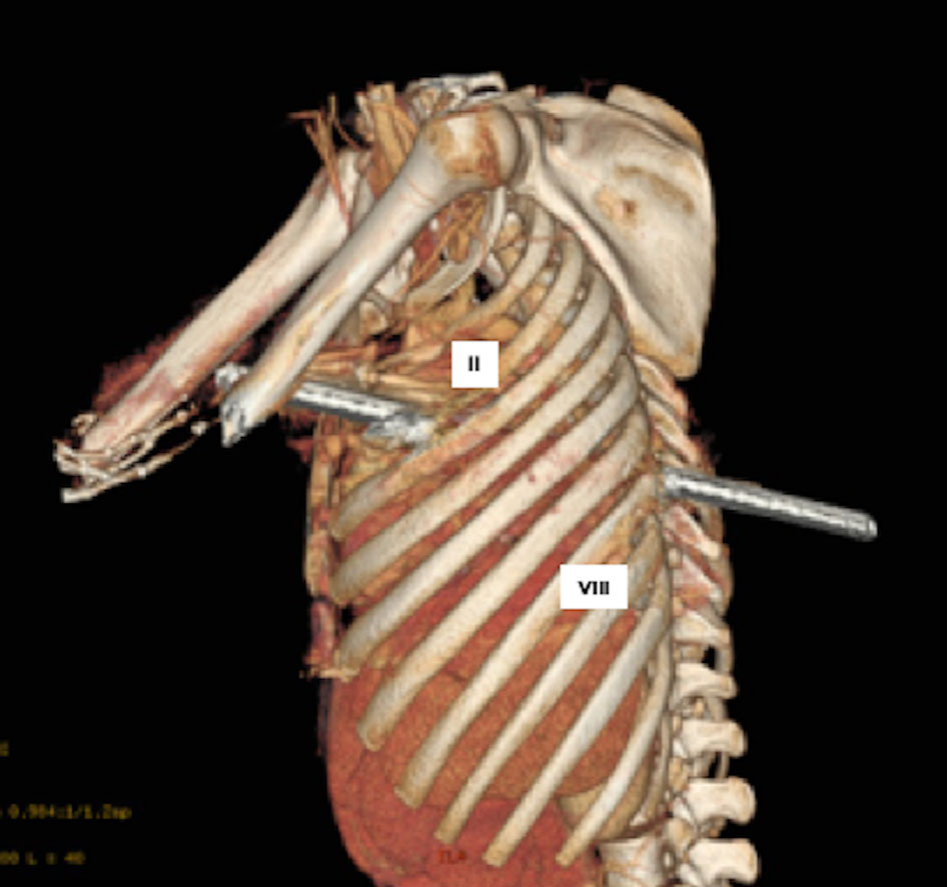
Three‐dimensional (3D) visualization of computed tomography (CT) scan reveals a rod penetrating left hemithorax through the eighth intercostal space of the posterior chest wall and coming out from the second intercostal space of the anterior chest wall. II, second intercostal space; VIII, eighth intercostal space.
